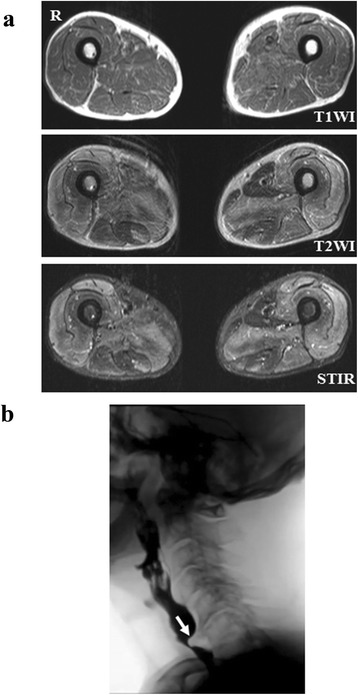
a Magnetic resonance imaging findings in the femoral muscles. T2-weighted and short tau inversion recovery magnetic resonance imaging scans show high signal intensity in both the flexors and extensors of the thigh muscles. b Videofluoroscopic study using paste barium. Pharyngeal muscle propulsion (cricopharyngeal bar) was observed (*arrow*)
